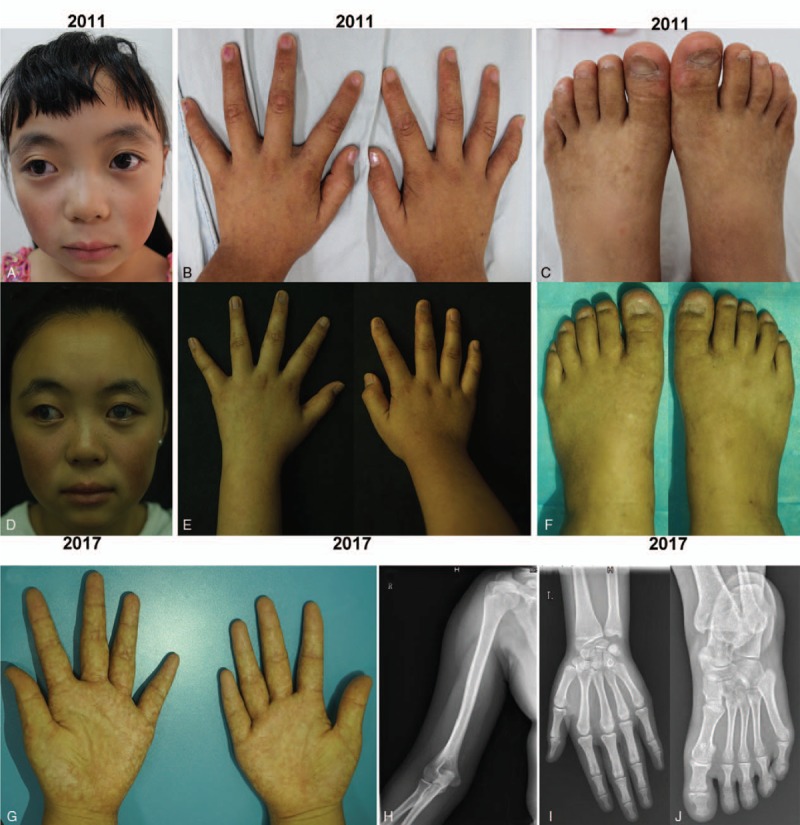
Facial dysmorphism, xerosis, and poikiloderma (A–C) were similar with her mother (Fig. 1A–C) when she was 7 in 2011. In addition, the daughter's toenails were malformed slightly (C) while shapes all the fingers and toes were roughly normal (B and C). At the age of 13 in 2017, though cutaneous lesions developed slowly (D–G), the patient's eyes were affected by emerging keratitis (D) while distal phalanges got thinner mildly (E–G) and nail abnormities, wrinkles on nails (E and F) and smaller toenails (F), were more marked. X-ray exanimations showed bowed humerus with thickened cortex (H–J) and distal phalangeal osteolysis.
